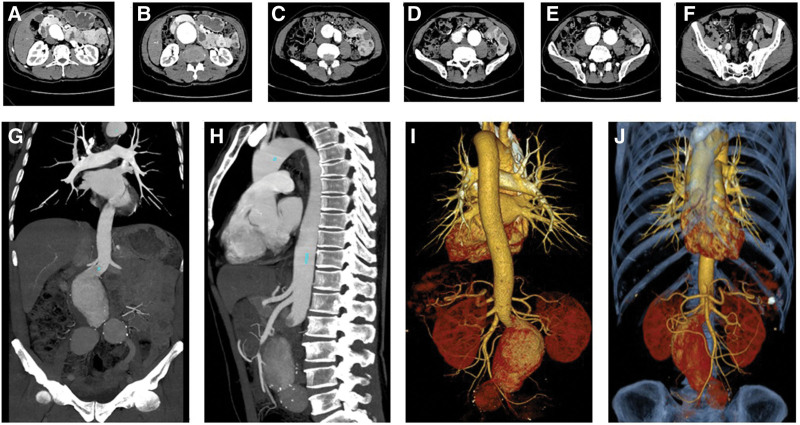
Abdominal vessels changes in enhanced CT findings. CT = computed tomography.
